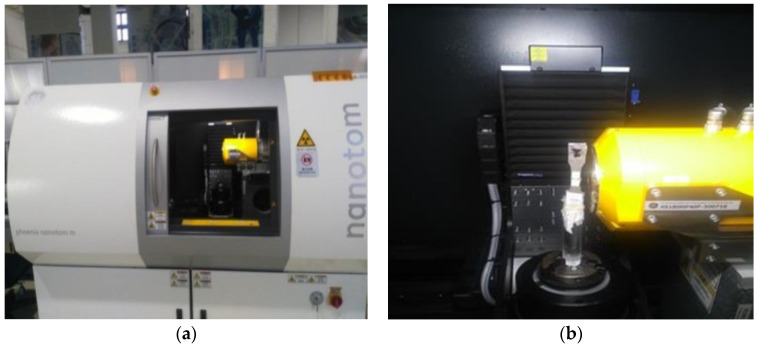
CT scanning device. (a) CT-Phoenix nanotom. (b) X-ray emitter.
